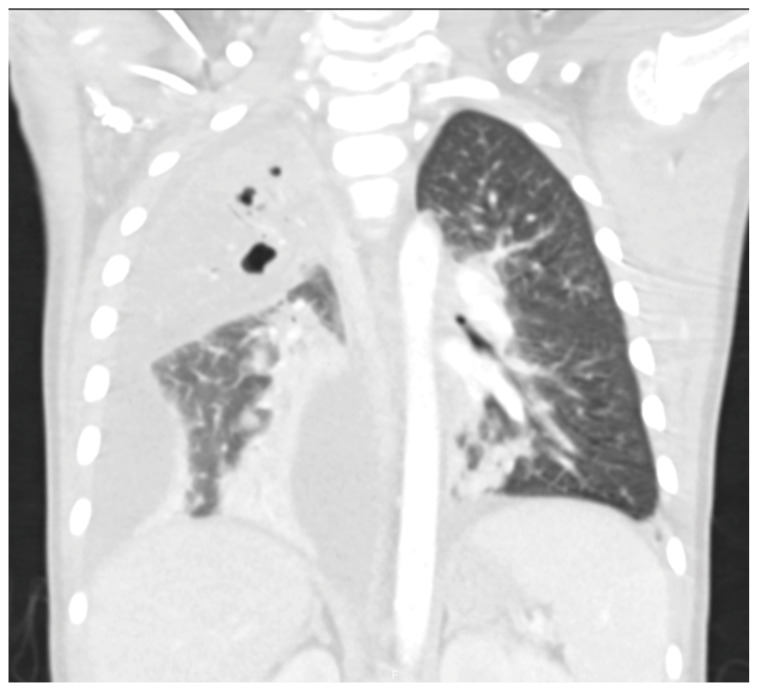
Necrotizing Pneumonia on Chest CT. Right upper lobg necrotizing pneumonia with cavitary changes and pneumotoceles. Moderate to large complex right parapneumonic effusion. Seen with lung windows.
